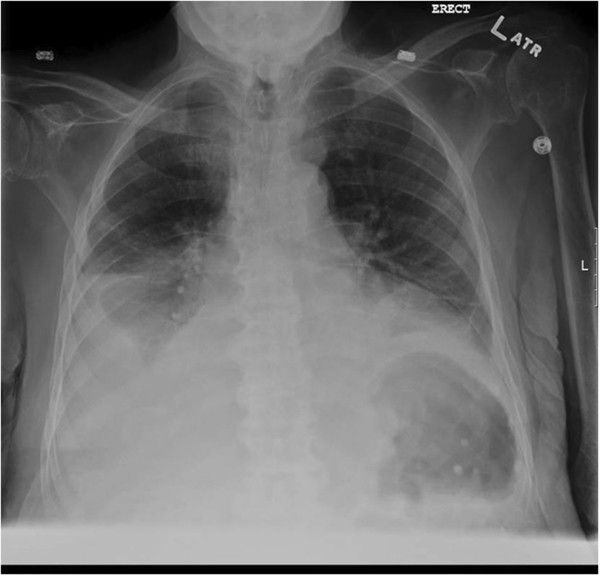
Chest X-ray showing elevated hemidiaphragms bilaterally with pleural effusions, perihilar edema and cardiomegaly.
